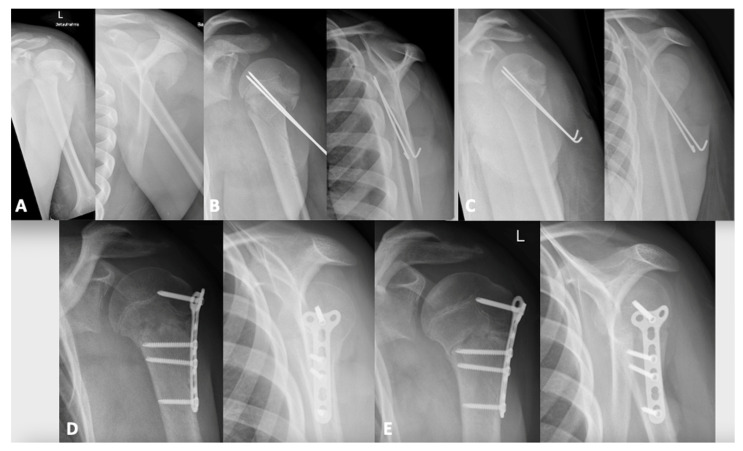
Chronologic radiographic images of Pat. N° 1. (A) Initial images after trauma with a posteriorly displaced proximal humeral fracture. (B) After closed reduction and transepiphyseal fixation. (C) X-ray 1 day after surgery shows posterior displacement in the Neer view (right side). (D) Open reduction and T-plate fixation. (E) X-ray follow-up 10 weeks after open reduction and plate-fixation with anatomical reduction and fracture healing.
